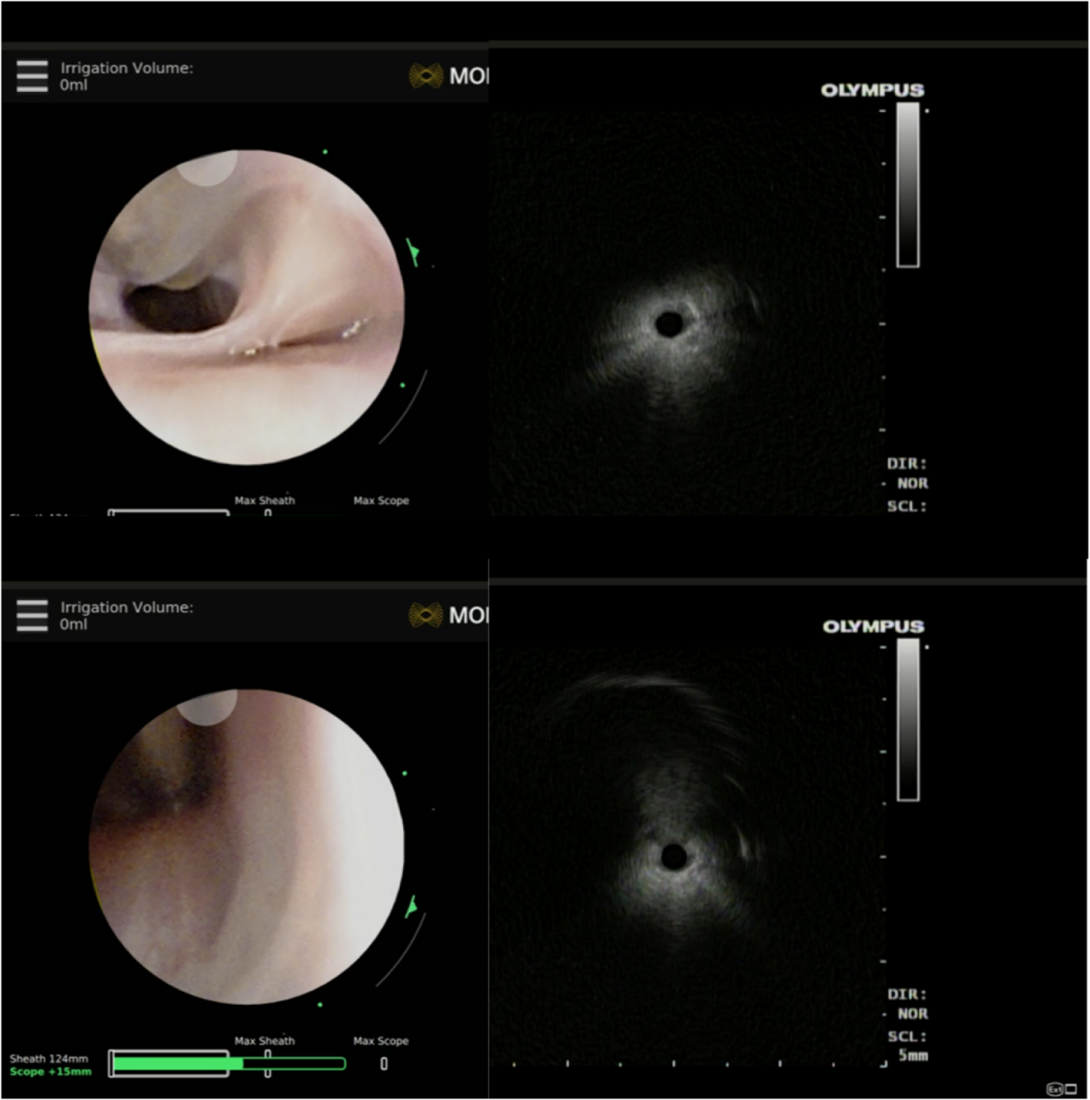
r-EBUS use to enable directional targeting of instruments. With RAB, an endoscopic view is maintained even in the smaller peripheral airways. Upper panel: the r-EBUS probe is in contact with the airway wall at the 11 o’clock position. The corresponding ultrasound image on the right shows only air artifact. Lower Panel: The r-EBUS probe is now directed to the 5 o’clock position of the airway wall and the ultrasound image reveals an eccentric view of the target lesion. An aspirating needle was oriented to penetrate the airway wall at the 5 o’clock position to obtain diagnostic tissue
